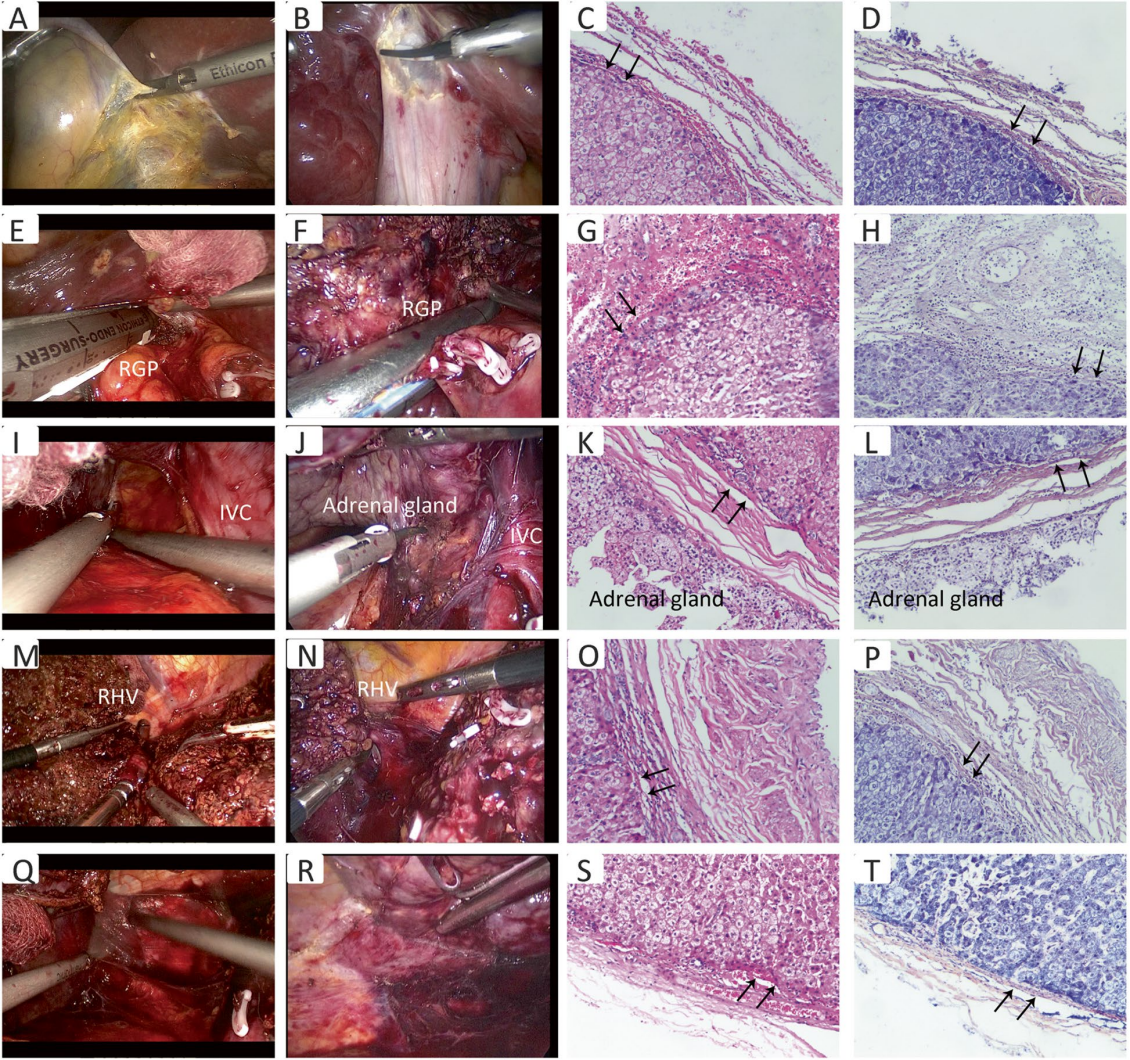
LARH via Laennec’s approach. A–D Laennec's approach was used to dissect the gallbladder. E–H The Goldfinger dissector was used to separate the GP of the right liver, and there was a gap between the GP and Laennec's capsule, and endo GIA stapler-cutter was used for the excision of the right liver pedicle. I–L The ultrasonic scalpel was used to separate the gap between the right liver and the right adrenal gland. M–P The gap between the right hepatic vein and the liver parenchyma was exposed, and then, endo-GIA stapler-cutter was used for excision. Q–T The ultrasonic scalpel was used to excise the perihepatic ligament, including the bare area. The arrows point to the Laennec’s capsule and magnified at 100×
